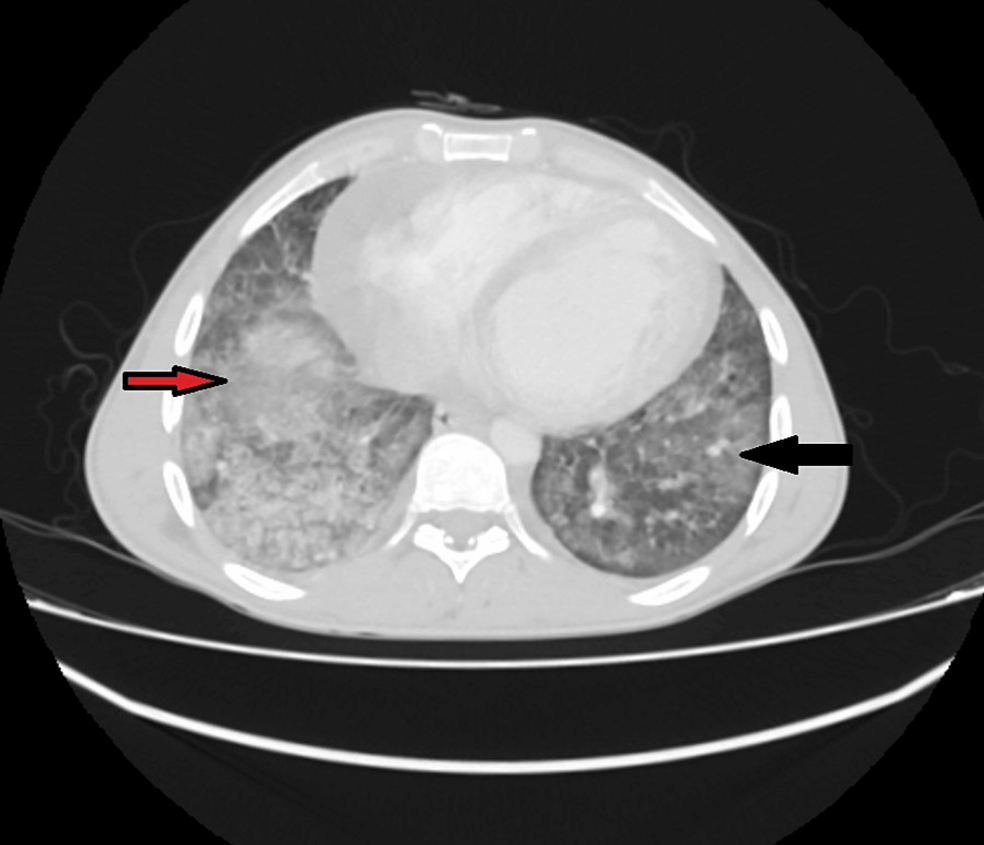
High-resolution CT scan showing bilateral dense multi-lobular shadowing on the background of ground-glass opacification with interlobular and intra-lobular septal thickening. Black arrow indicates ground-glass opacities. Red arrow indicates consolidation in the background of -round glass opacities.
